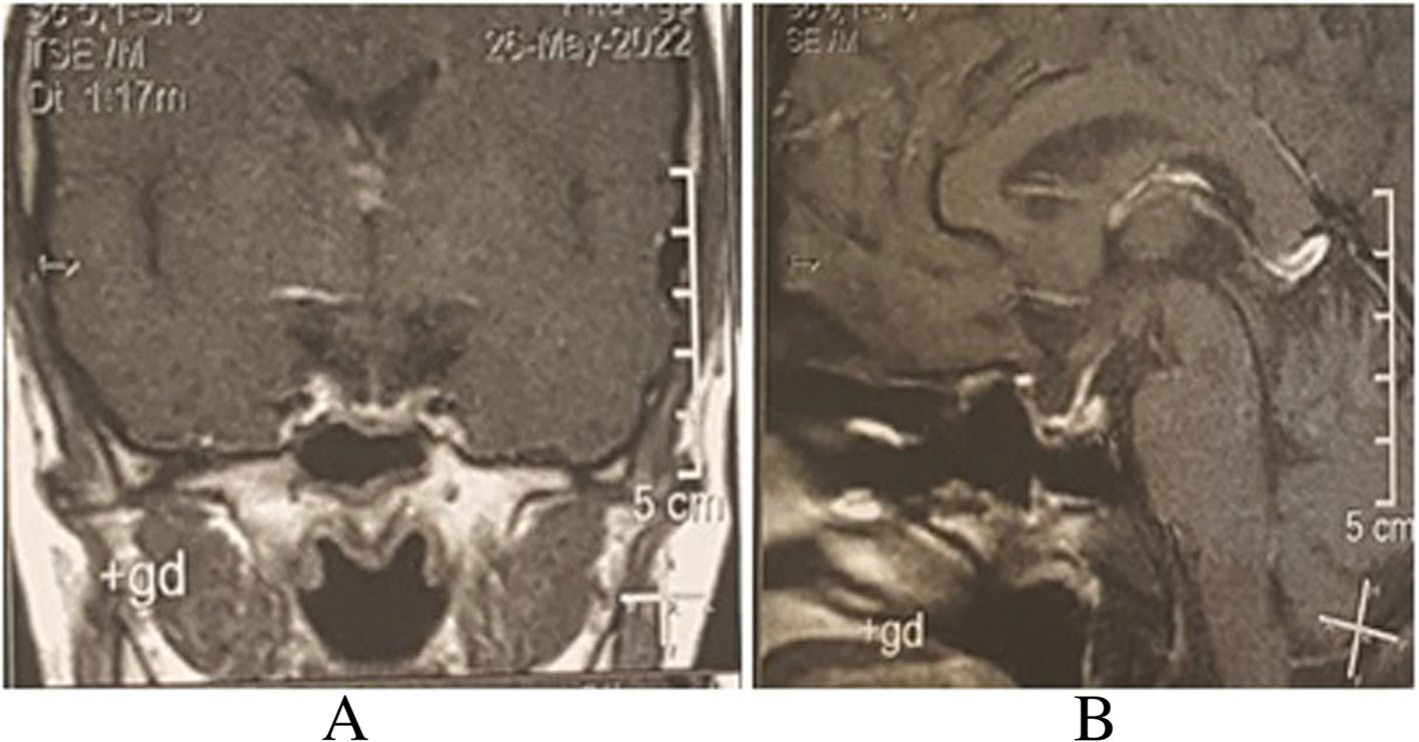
A, B Sagittal and Coronal post contrast T1-weighted of pituitary gland demonstrate normal pituitary gland remnant without stalk thickening or superior convexity
